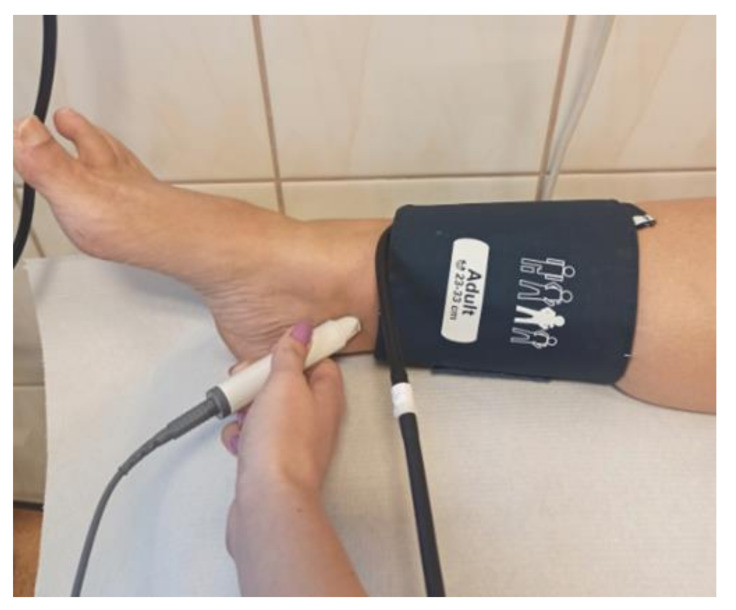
Doppler ABI measurement.
